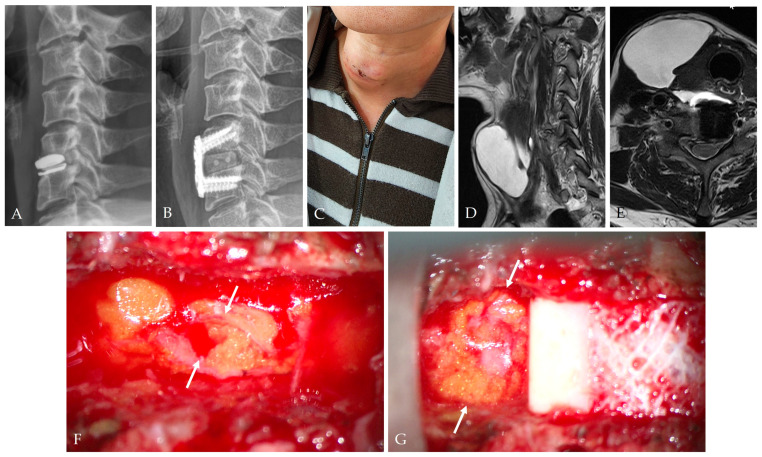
A 51-year-old female patient underwent C5–6 ACDF for failure of cervical disc arthroplasty (CDA) (A,B). At postoperative 2 weeks, the patient complained of anterior right side neck swelling (C). Follow-up MRI showed an extensive CSF collection (D,E). During the revision surgery, a dural tear (white arrows) was identified (F), and fat graft (white arrows) was applied to close the CSF leakage site (G).
